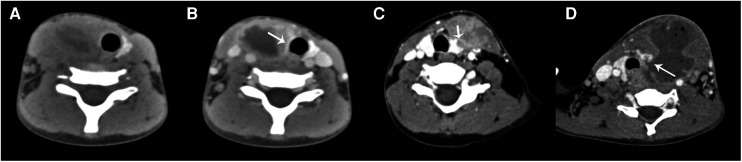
(A,B) A 4-year-old girl had a right neck mass for 5 days. (A) CT plain scan showed a soft tissue mass in front of the right neck with necrotic lesions. (B) Contrast-enhanced CT showed abscess wall thickening and annular enhancement; the right lobe of the thyroid presented a low-density shadow and unclear boundary (arrow). The trachea and carotid sheath were displaced. (C) A 6-year-old boy had a left neck mass for 14 days. Contrast-enhanced CT showed a soft tissue mass in the front of the left neck with uneven enhancement; the left lobe of the thyroid was involved with the low-density shadow extending to the mass (arrow). (D) A 9-year-old boy had a left neck mass with a fever for 4 days. Contrast-enhanced CT showed the formation of a left anterior cervical abscess, accompanying gas accumulation, displacement of trachea and carotid sheath, and stenosis of the left internal jugular vein; the left lobe of the thyroid and the posterior pharyngeal wall were involved with the low-density shadow extending to the abscess (arrow).
